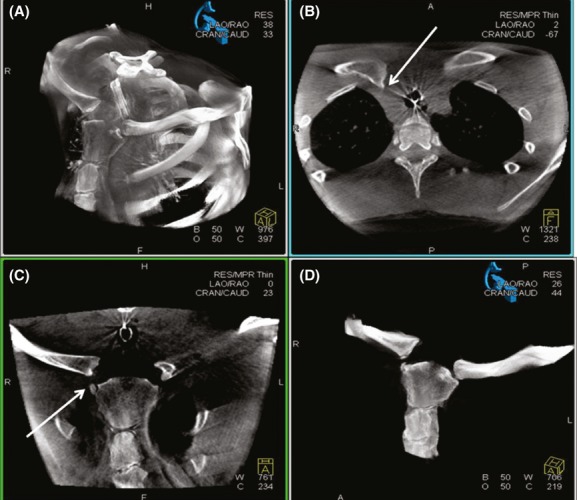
Cone beam computed tomography (CT) performed in the hybrid theatre demonstrating adequate reduction in the sterno-clavicular joint. The above images demonstrate the anatomy from the cone beam CT scan immediately after closed reduction in the posterior sterno-clavicular dislocation. (A) An overall volume-rendered 3D image reconstruction of the anatomy. (B) An axial plane reconstruction and how the medial end of the clavicle is no longer compressing the trachea (arrow). (C) Coronal reconstruction demonstrating a small avulsion fracture of the lateral aspect of the sternum (arrow). (D) An oblique 3D reconstruction demonstrating improved alignment of the clavicle in relation to the sternum.
